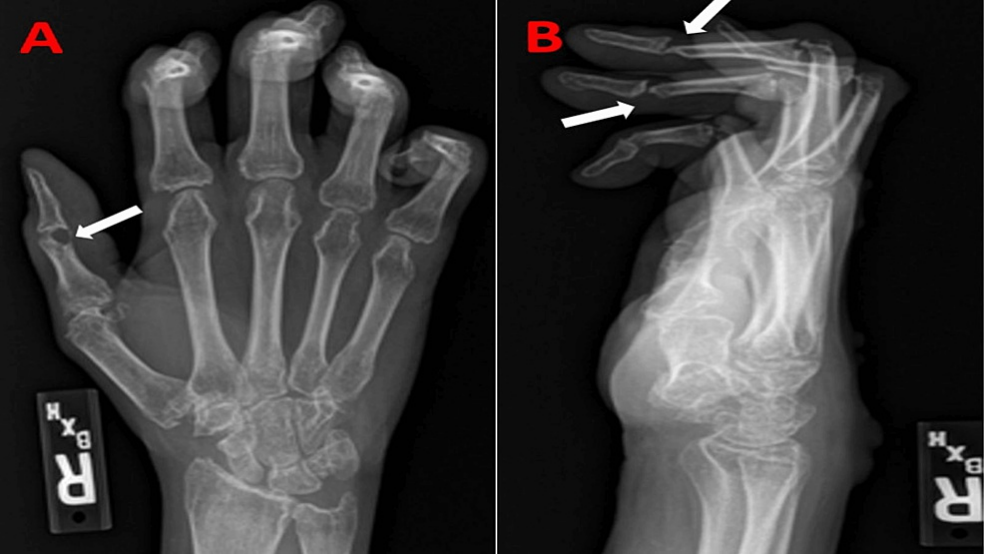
X-ray right hand posteroanterior (A) and lateral (B) views demonstrating severe bone destruction around the interphalangeal joints (white arrows).
